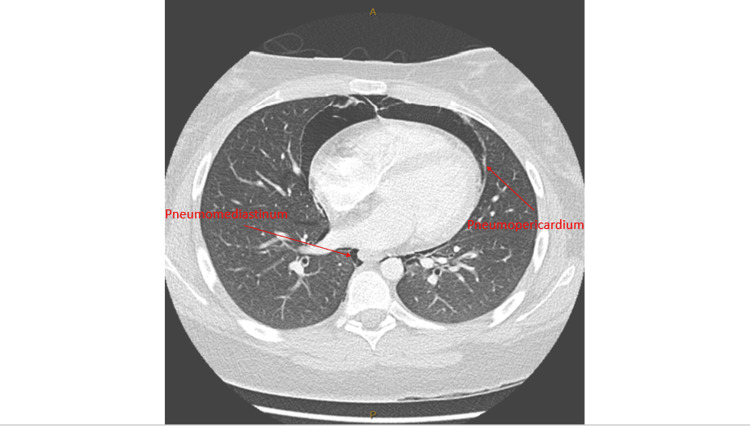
Axial view of CT chest demonstrating pneumomediastinum and pneumopericardium.
